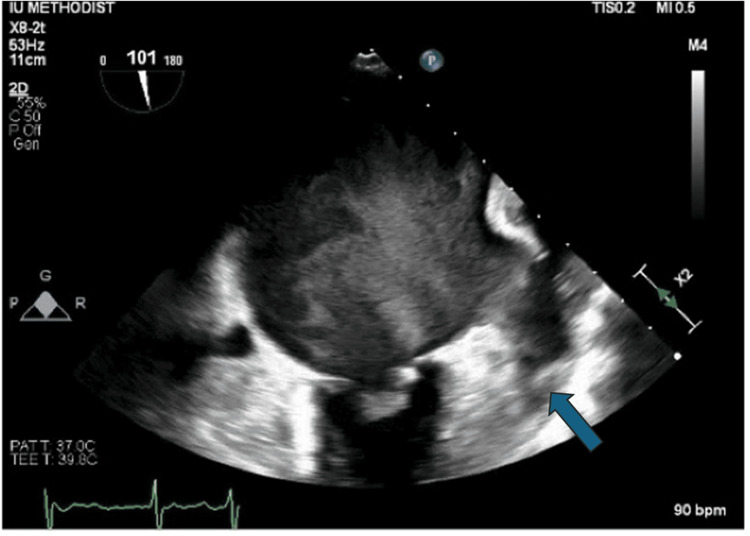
Transesophageal echocardiography image demonstrating an echodense mass within the left atrial appendage (blue arrow), concerning for thrombus
